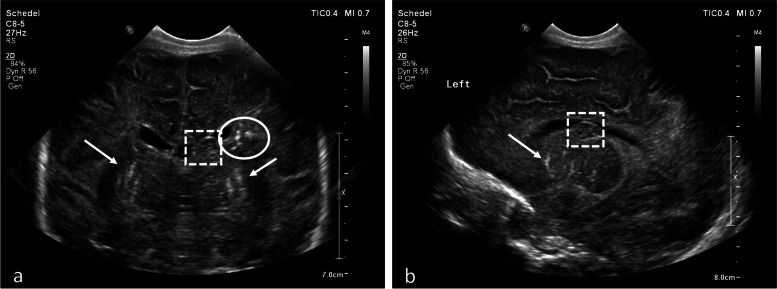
Postnatal cranial ultrasound, convex transducer. a coronal plane at the foramen of Monro. b parasagittal plane over the left ventricle. At day 1 of life lenticulostriate vasculopathy (white arrow), germinolysis (white square) and extensive periventricular calcifications (white circle) were visible
